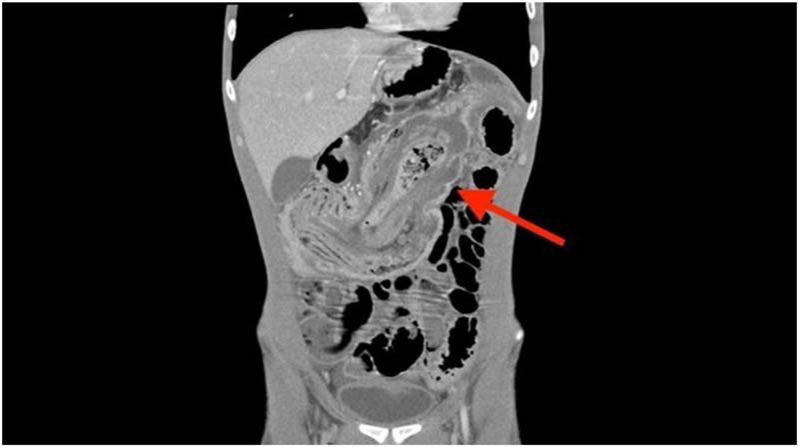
Coronal CT scan showing ileocolonic intussusception extending to the sigmoid colon.
